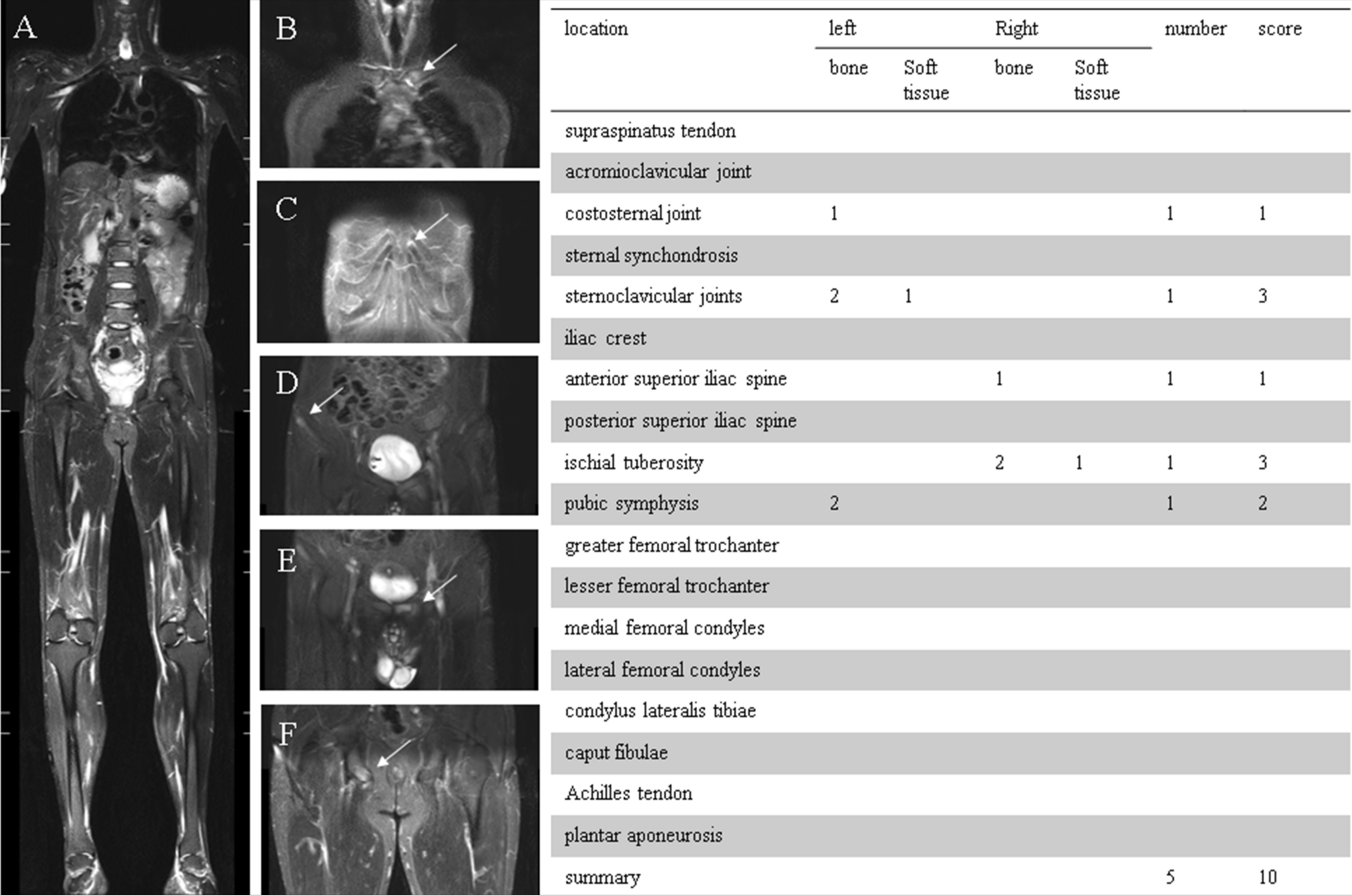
(A) 36-year-old man, HLA-B27 positive, was classified as ankylosing spondylitis (AS). (A) Coronal STIR whole-body MRI image demonstrated entheseal sites from shoulder to heel. (B) Enthesitis of the left sternoclavicular joint (including bone marrow edema and joint effusion). (C) Enthesitis of the left costosternal joint. (D) Enthesitis of the right anterior superior iliac spine (bone marrow edema). (E) Enthesitis of the pubic symphysis (bone marrow edema). (F) Enthesitis of the right ischial tuberosity (including bone marrow edema and soft tissue inflammation). The table on the right indicates the numbers and scores of entheses.
